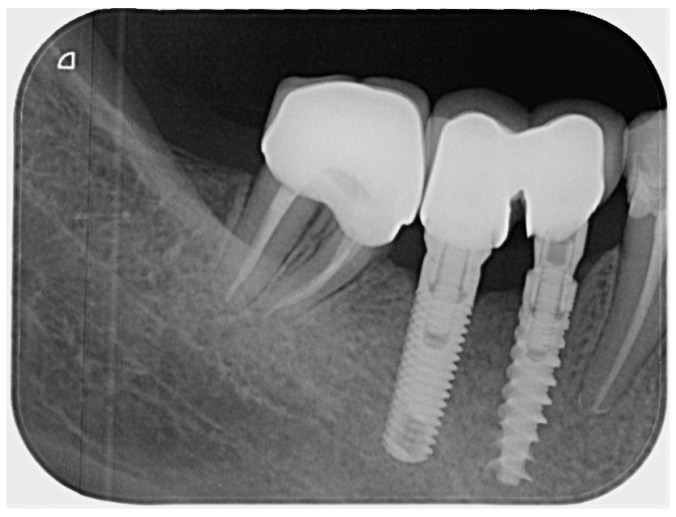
Control periapical radiograph of tooth 47 taken 2.5 years after completion of endodontic and prosthetic treatment.
